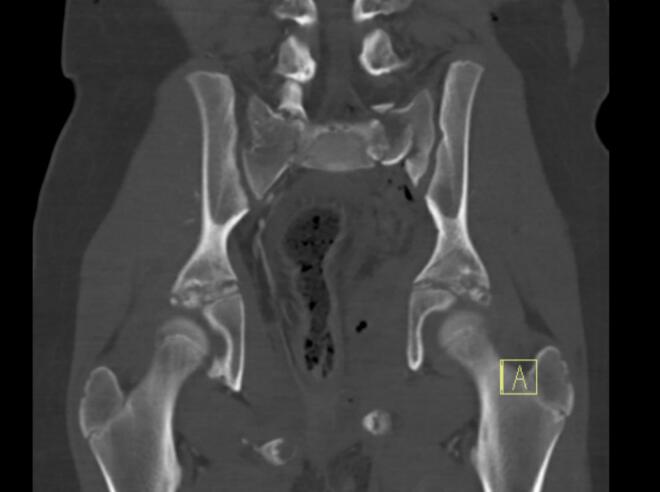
Coronal view of CT-scan of a 12 year old female who sustained a Tile type B3 Pelvic fracture.
